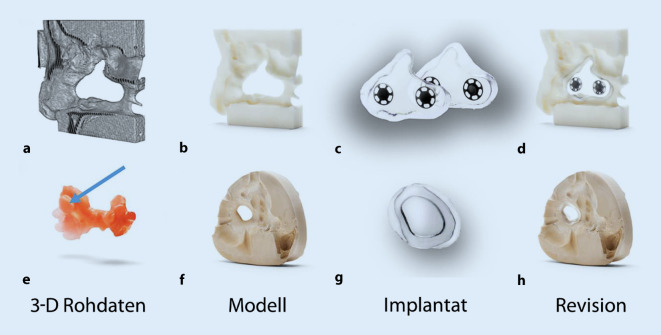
Alle Methoden haben jedoch Nachteile aus Patientensicht: Konfektionierte Implantate sind nicht patientenspezifisch und haben eine schlechte Passung, die zu Krustenbildungen führen kann. Bei sehr großen Defekten sind Silikonabformungen aufgrund fehlender Auflagepunkte größenlimitiert, außerdem erfordern sie eine Betäubung und können zu Verletzungen führen (erhöhtes Morbiditätsrisiko). CT-Daten stehen im Vergleich dazu im Konflikt mit der Strahlenhygiene. Jedes Verfahren erfordert unabhängig davon eine anatomische Vermessung der meist nicht kreisförmigen Perforation. Typische Perforationen können von wenigen Submillimetern bis zu mehreren Zentimetern reichen. Bei der Größenbestimmung ist es zudem wichtig, die vertikale als auch die horizontale Länge der Perforation zu messen (Abb. 3). Insbesondere die vertikale Perforationshöhe spielt eine Schlüsselrolle für den Therapieerfolg, da sie unmittelbare Auswirkungen auf die Spannung zwischen Nasenboden und Nasenrücken hat [4, 9]. Zudem ist eine spätere chirurgische Behandlung nicht ausgeschlossen. Für chirurgische Behandlungen sind mehrere Techniken mit unterschiedlichen Erfolgsraten beschrieben. Sie beruhen auf 2 Grundprinzipien: der Verwendung von Schleimhautlappen unddem Einsetzen eines Interpositionselements zwischen den beiden Schleimhautoberflächen [6, 14, 20–23].
